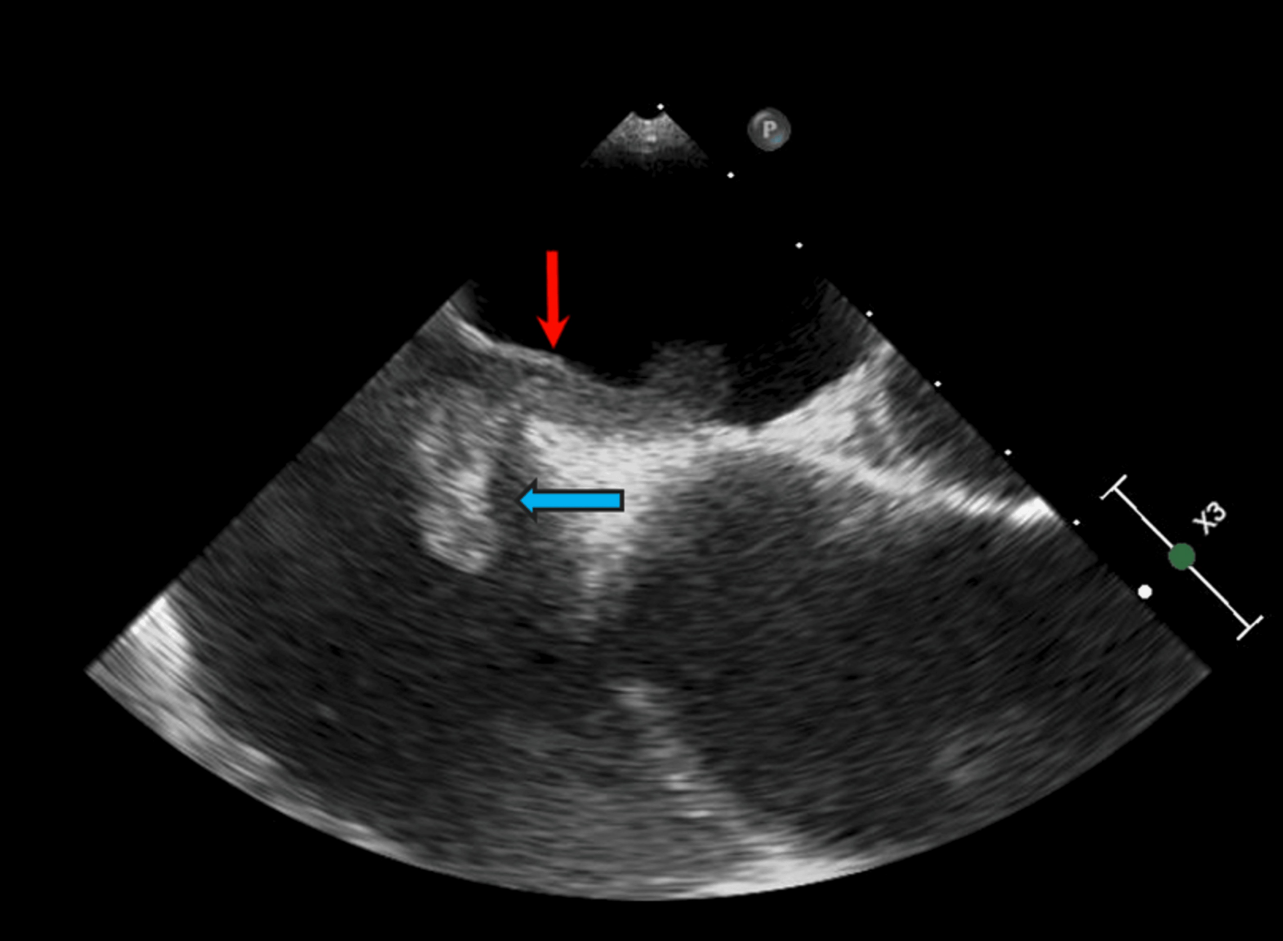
TEE in the mid-esophageal four chamber view showing a TIT measuring approximately ten centimeters in length across a PFO. The red arrow shows PFO and blue arrow shows the clot. This TIT required thrombectomy to prevent systemic embolism. TEE: transesophageal echocardiography; TIT: thrombus in transit; PFO: patent foramen ovale.
